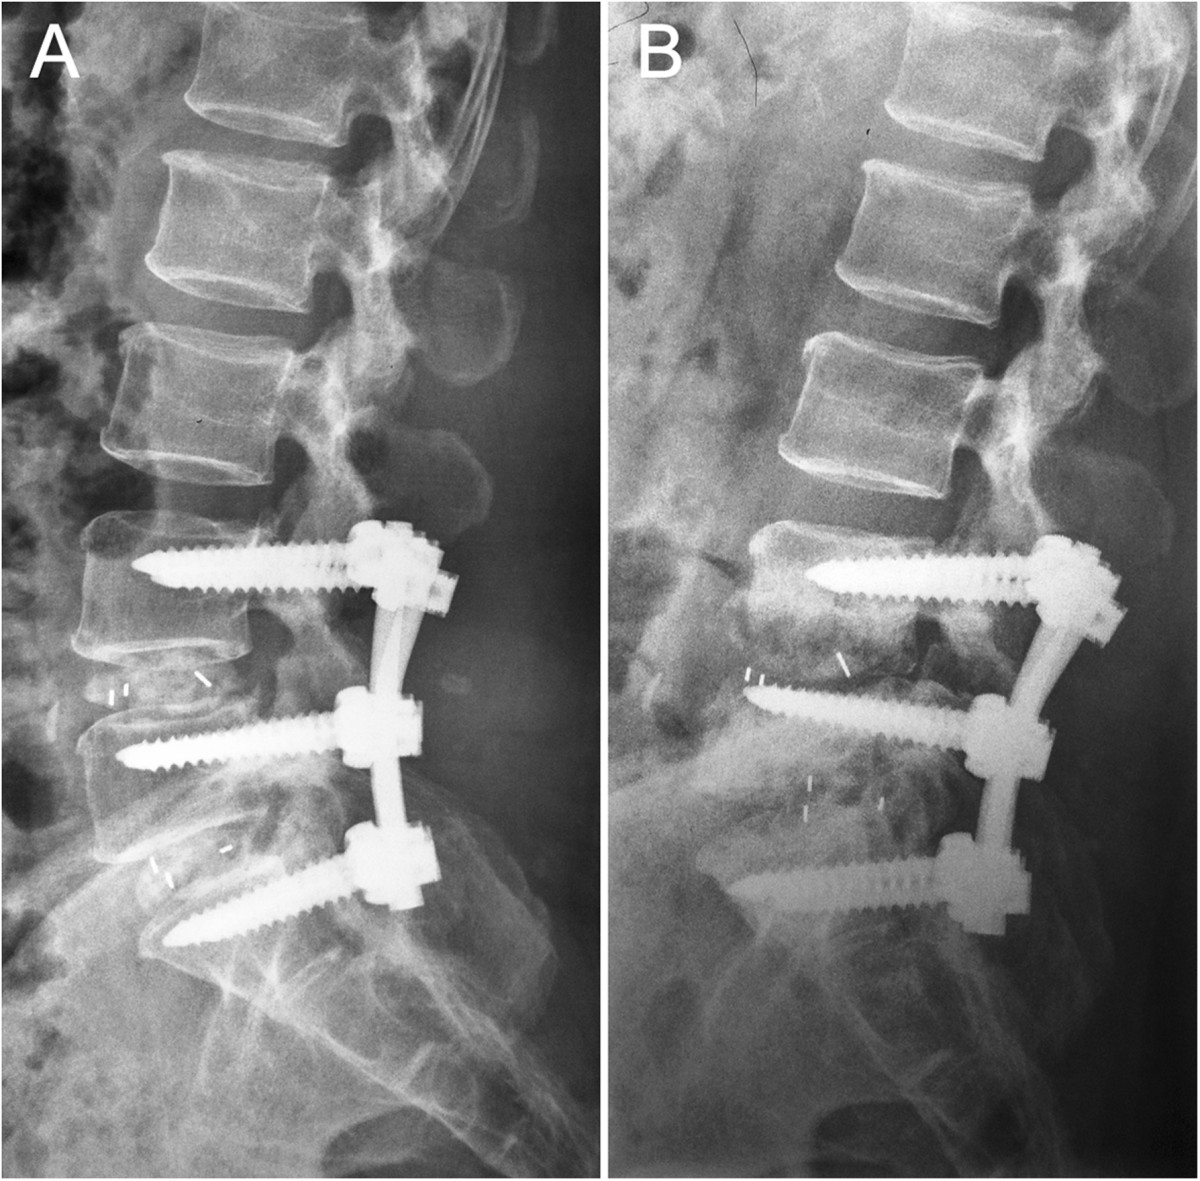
Lateral radiographs of the internal fixator and spine. (A) An immediate postoperative lateral radiograph. (B) Radiograph at 3 mo after postoperative, showing areas of osteolysis and malposition of the pedicle screws.
